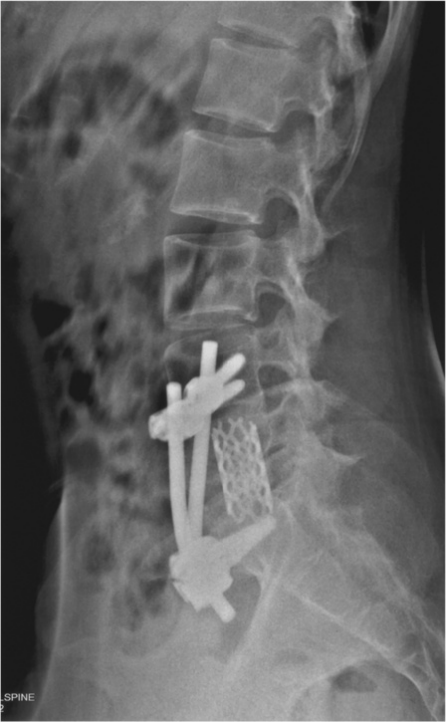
Postoperative lateral radiograph of screw-rod construct fixation underneath the iliac vessels in combination with one-stage anterior debridement and fusion for lumbosacral spinal tuberculosis
